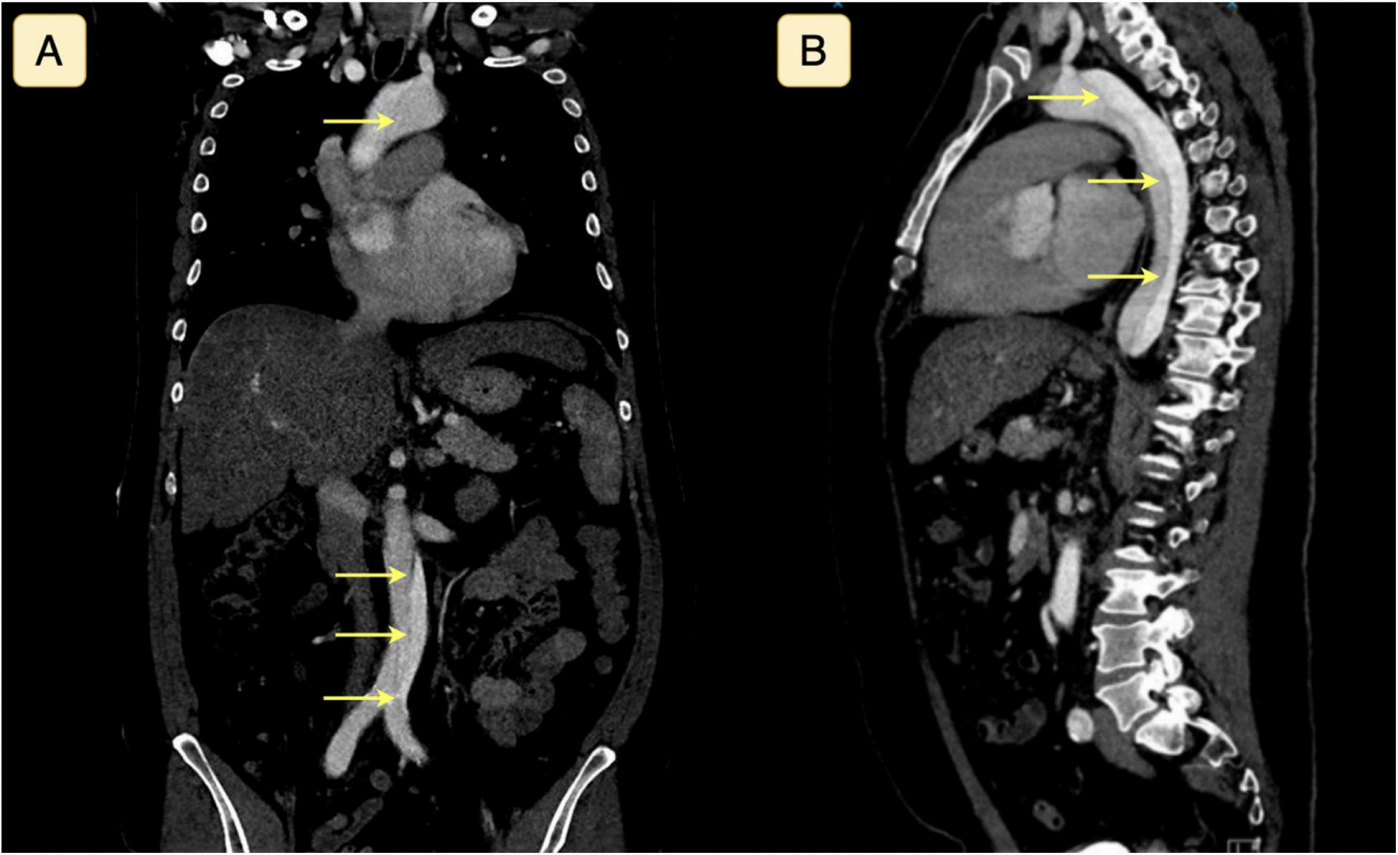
Computed tomography with angiography of the thorax and abdomen seen in coronal (A) and sagittal (B) plane, demonstrating a Stanford type A aortic dissection. The yellow arrows highlight the dissection plane separating the true and false lumina.
